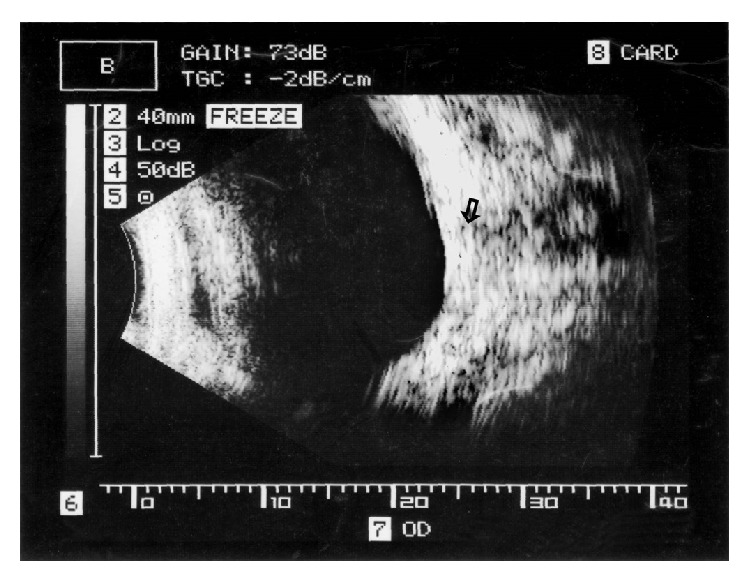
Vertical macula B-scan ultrasonogram demonstrating a calcific subretinal focus (arrow) with acoustic aftershadow of retrobulbar fat.
